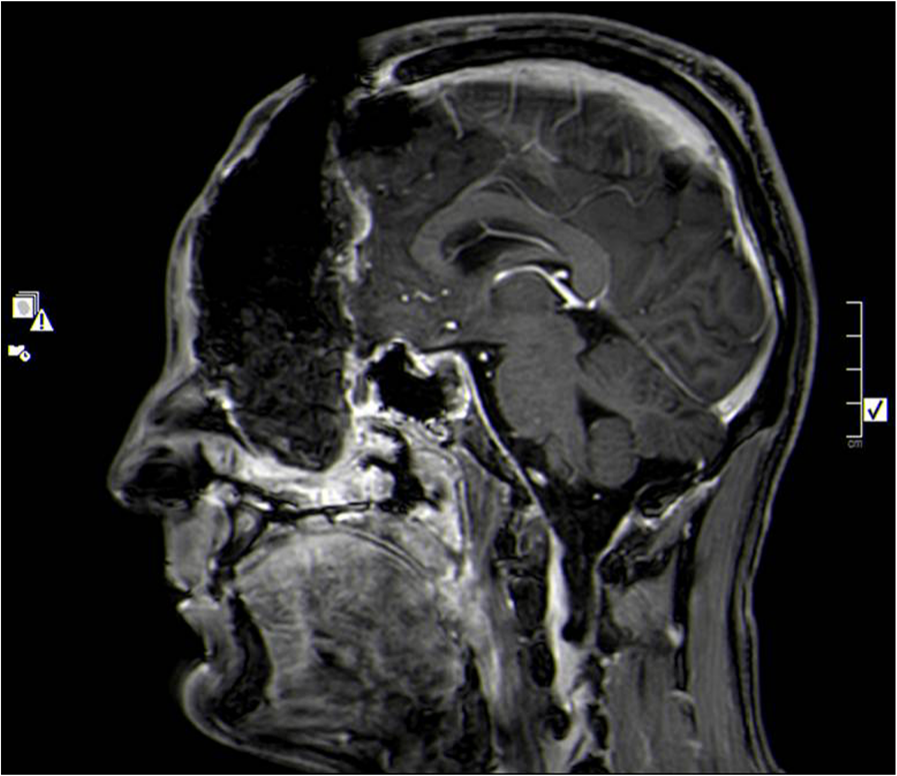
Brain MRI. Sagittal view. Post-contrast T1 weighted image. Showing post-operative cavity without gross residual mass
